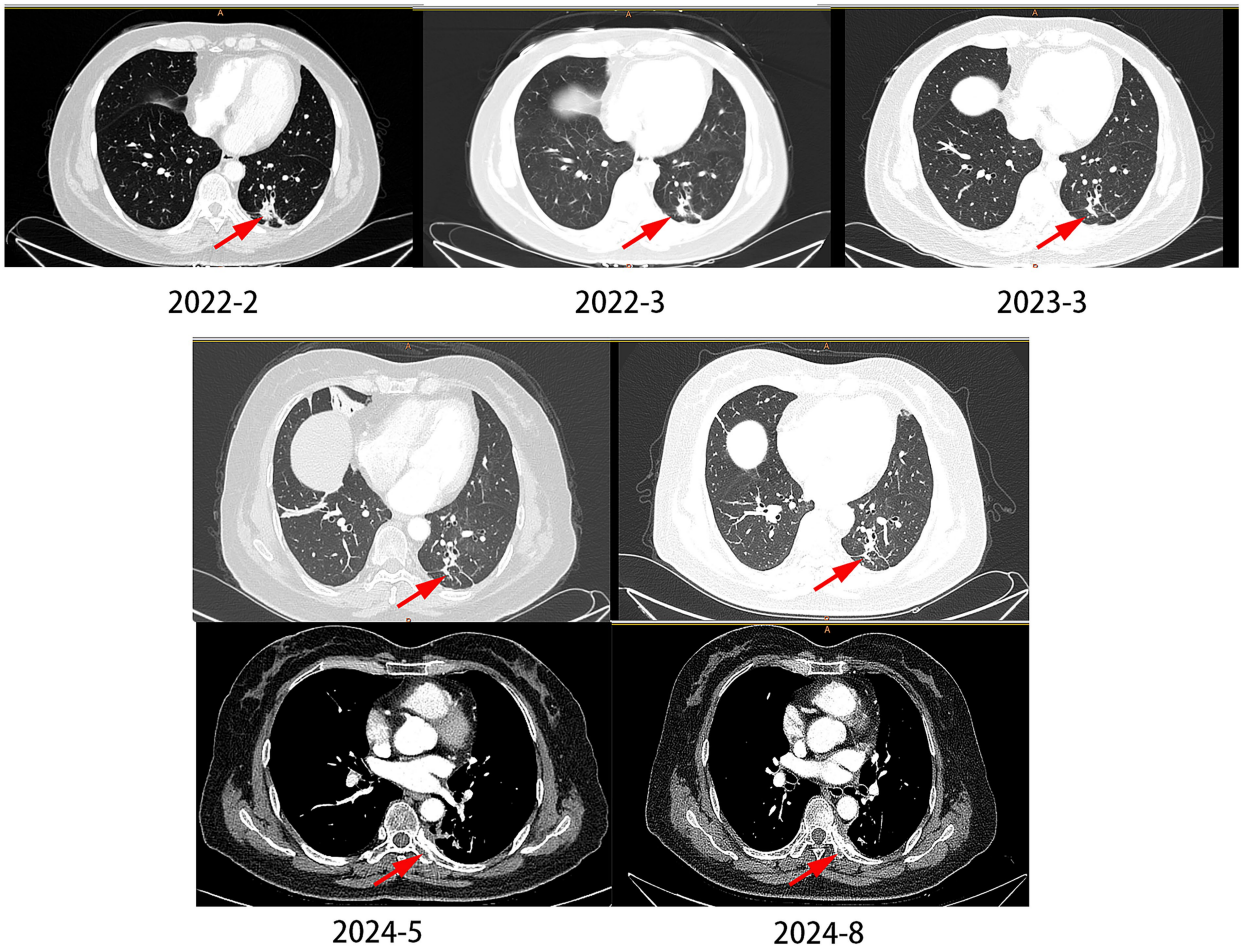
The changes of lung CT scan in case 2. In 2024–2028, the patient developed bone metastases, but the pulmonary nodules did not significantly enlarge.
